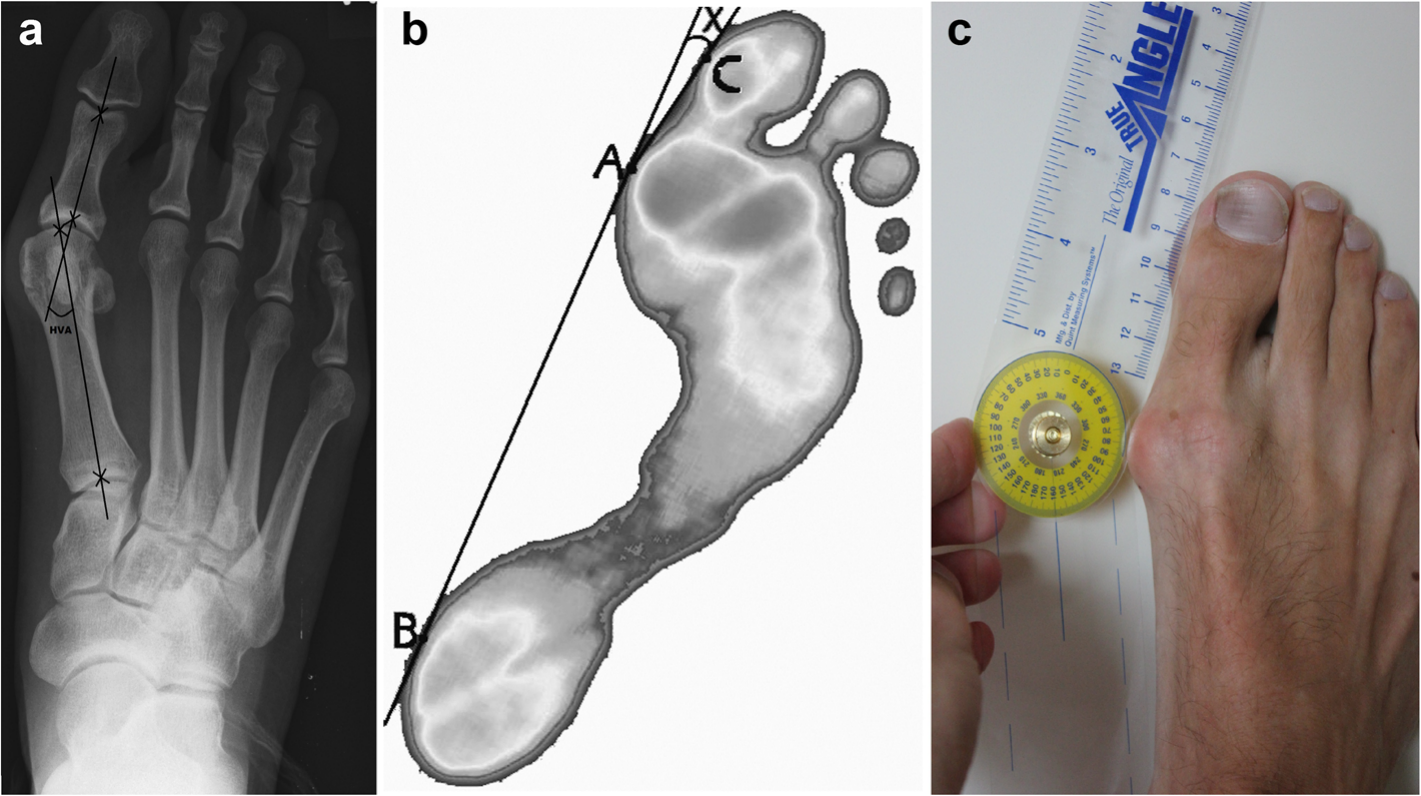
HVA measurement through radiography (a) according to the method of Mitchell et al., through computerised plantar pressure (b) and through clinical examination (c).
